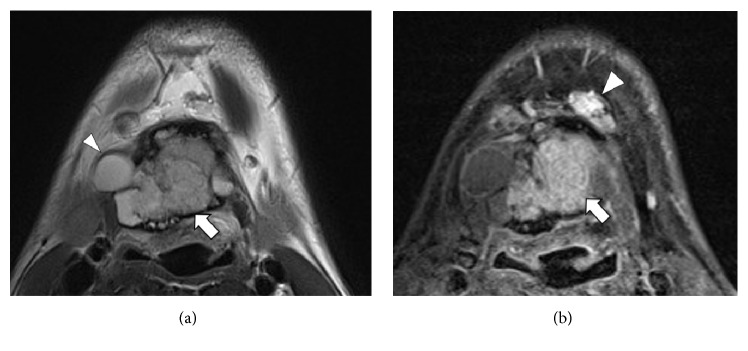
MRI of the neck. (a) T2-weighted image at the level of the hyoid bone shows intermediate signal intensity corresponding to the solid component (arrow) and high signal intensity corresponding to the cystic component (arrowhead). (b) Contrast-enhanced fat-suppressed T1-weighted image at the same level as (a) shows marked enhancement of the solid component (arrow). Left submental lymphadenopathy is also seen (arrowhead).
